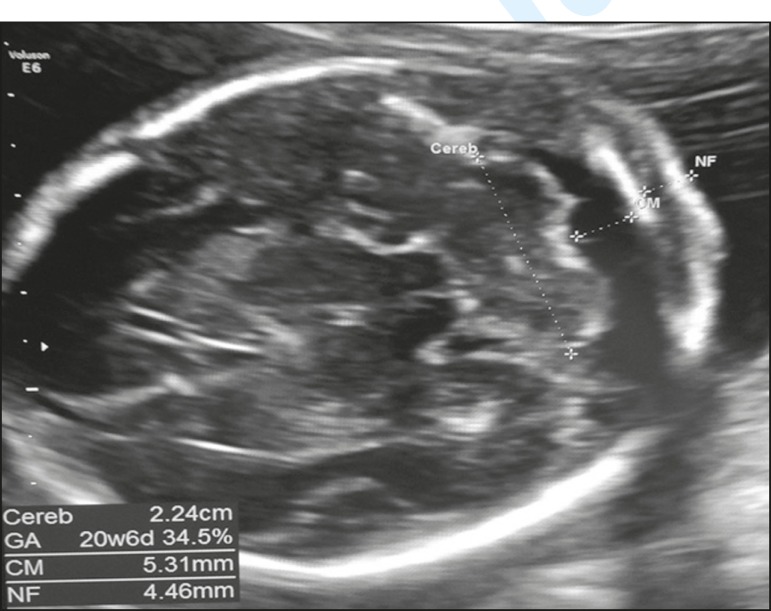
Axial ultrasound of the transcerebellar plane, evaluating the cerebellar hemispheres (shape and contours); the vermis (more highly echogenic structure between the two cerebellar hemispheres); the biometry of the cerebellum (transcerebellar diameter); the shape and transverse diameter of the cisterna magna; and the size of the fourth ventricle.
